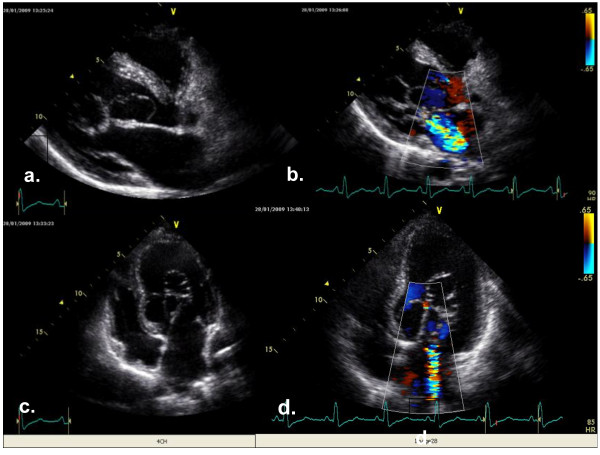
Parasternal long axis and four-chamber view of an unusual presentation of a mitral valve. (a) Parasternal long axis view shows chordae meshwork into the left ventricle cavity. (b) Parasternal long axis view of color Doppler shows moderate to severe mitral regurgitation. (c) Four-chamber view shows elongated chordae that were predominantly oriented to the septum. (d) Four-chamber view of color Doppler confirmed the presence of moderate to severe mitral regurgitation.
